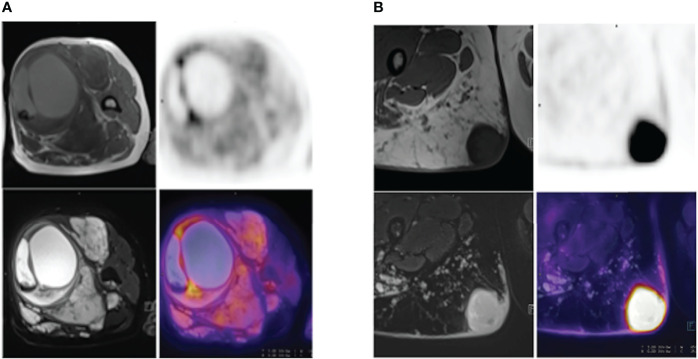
(A) A 52-year-old NF1 patient with benign PNF lesions, particularly in the left thigh. The lesion was located in the soft tissue of the upper segment of the left thigh, was approximately 8.7 cm-9.5 cm in size, with clear boundary, heterogeneous internal signal, visible cystic degeneration with hemorrhage, and increased FDG uptake at the septa, SUVmax=4.9. (B) A 28-year-old NF1 patient with a MPNST in the upper segment of the right thigh, measuring 3.9 cm – 3.8 cm, with well-defined borders and increased FDG uptake, SUVmax=14.4.
